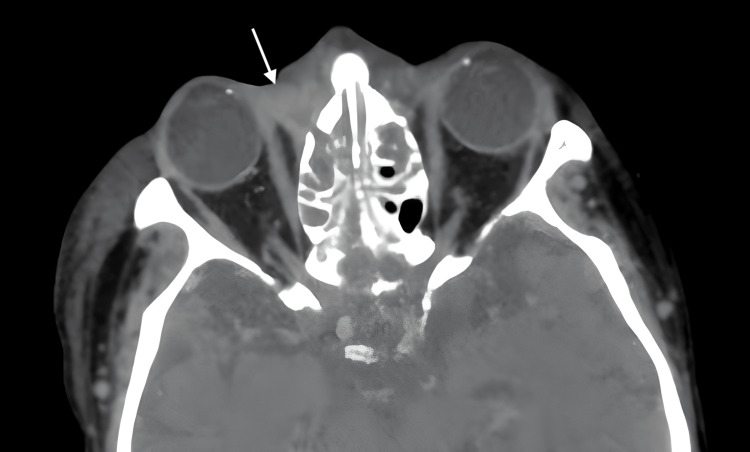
Pre-operative axial CT of periorbital space Preseptal enhancement within the periorbital space (white arrow) was also observed on imaging shortly after admission.
